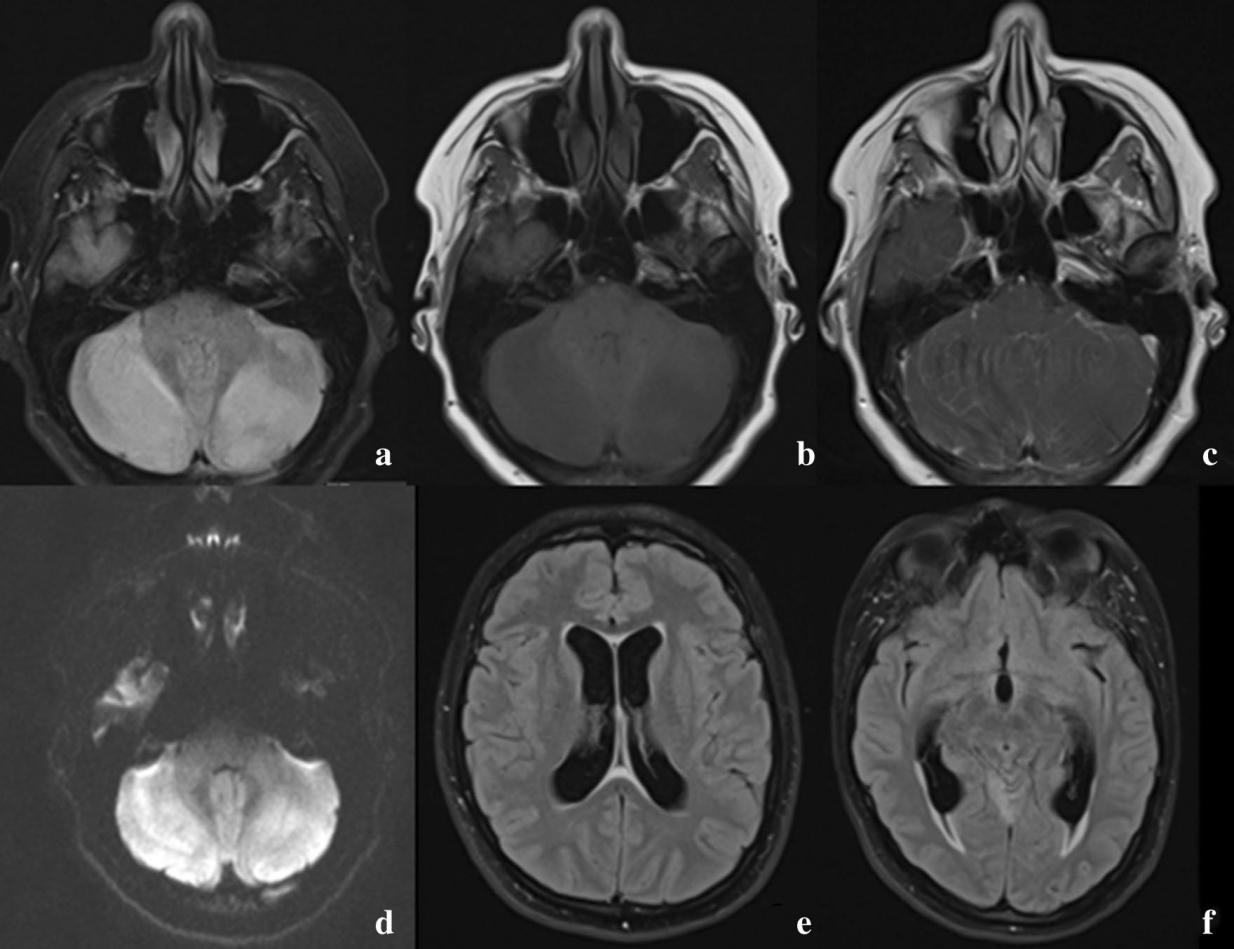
Brain magnetic resonance imaging (MRI) of our case, consistent with cerebellitis and hydrocephalus. From left to right, up to down: a FLAIR sequence, showing hyperintense cerebellar hemispheres. b T1 sequence, showing hypointense cerebellar hemispheres. c T1 sequence with gadolinium, showing leptomeningeal enhancement of the cerebellum. d Diffusion sequence, showing restrictive diffusion of the cerebellar hemispheres. e FLAIR sequence, showing enlarged lateral ventricles. f FLAIR sequence, showing enlarged temporal horns with transependymal oedema, as sign of increased intraventricular pressure
